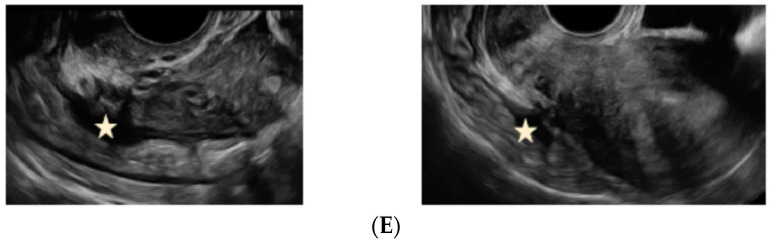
The first step was to recruit the endometriosis patients diagnosing them by expert sonographers with transvaginal ultrasound. 3D-transvaginal ultrasound images of different locations of deep endometriosis taken from our Endometriosis Unit at the Hospital Clínic of Barcelona ((A): bladder endometriosis nodule; (B): uterosacral ligament endometriosis nodule; (C): rectum wall nodule; (D): vagina wall nodule; (E): posterior compartment uterosacral ligament and rectum endometriosis nodule marked with an asterisk).
